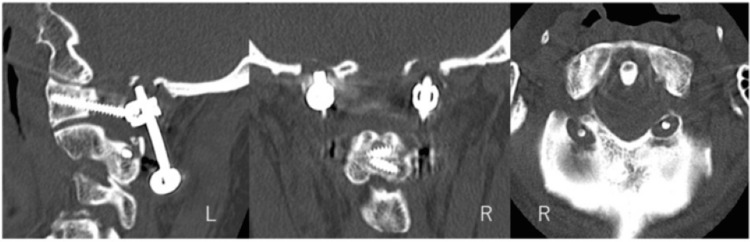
Multiplanar reconstruction computed tomography (MPR-CT) Unveiling bilateral remarkable bony erosion around the C1LMS-R-Con (C1LMS-R-Con, C1 lateral mass screw-rod construct; R, right side; L, left side).
